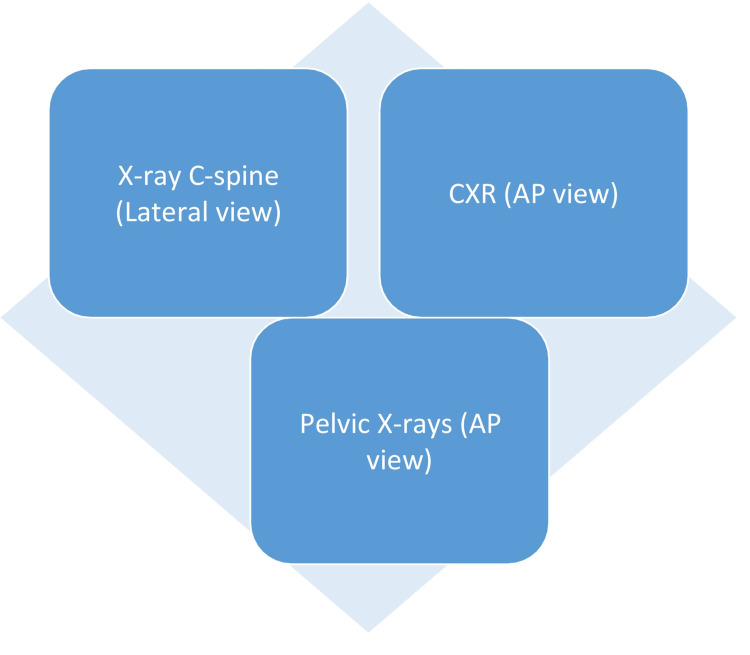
Trauma Series C-spine = cervical spine; CXR = chest X-ray; AP = anteroposterior
